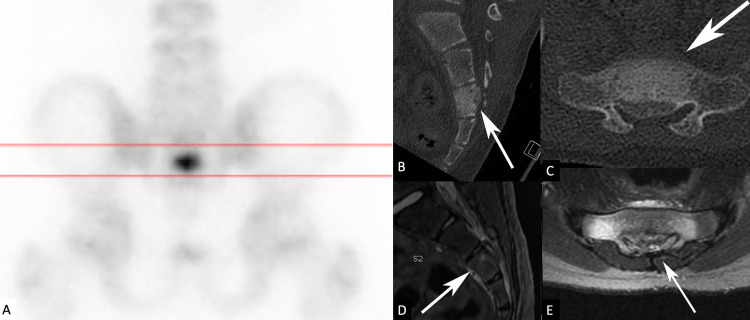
Bone scan (A) showing increase captation in the sacral region. CT scan (B, C) and MRI (D, E) showing recurrence of the bony lesion with periosteal reaction (arrows)
